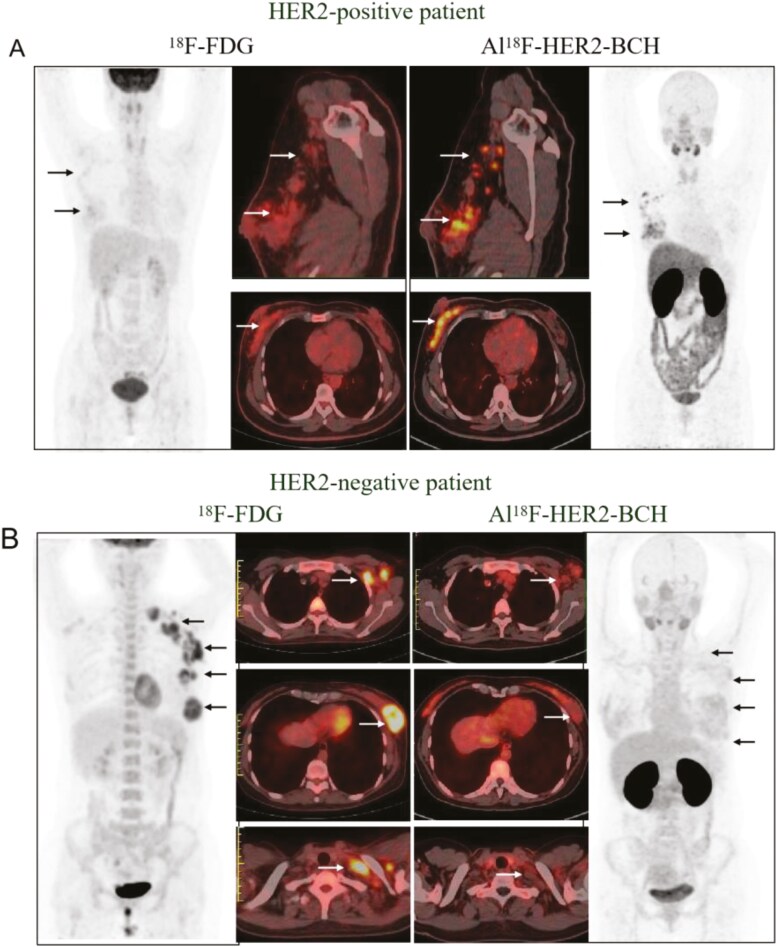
Images of ^18^F-FDG PET/CT and Al^18^F-NOTA-HER2-BCH PET/CT in a HER2-positive and HER2-negative patient. (A) A 47-year-old patient with primary diffuse breast cancer and multiple lymph nodes. Biopsy proved IHC 3+. (B) A 39-year-old with recurrence and metastasis. Biopsy proved IHC 1+.
